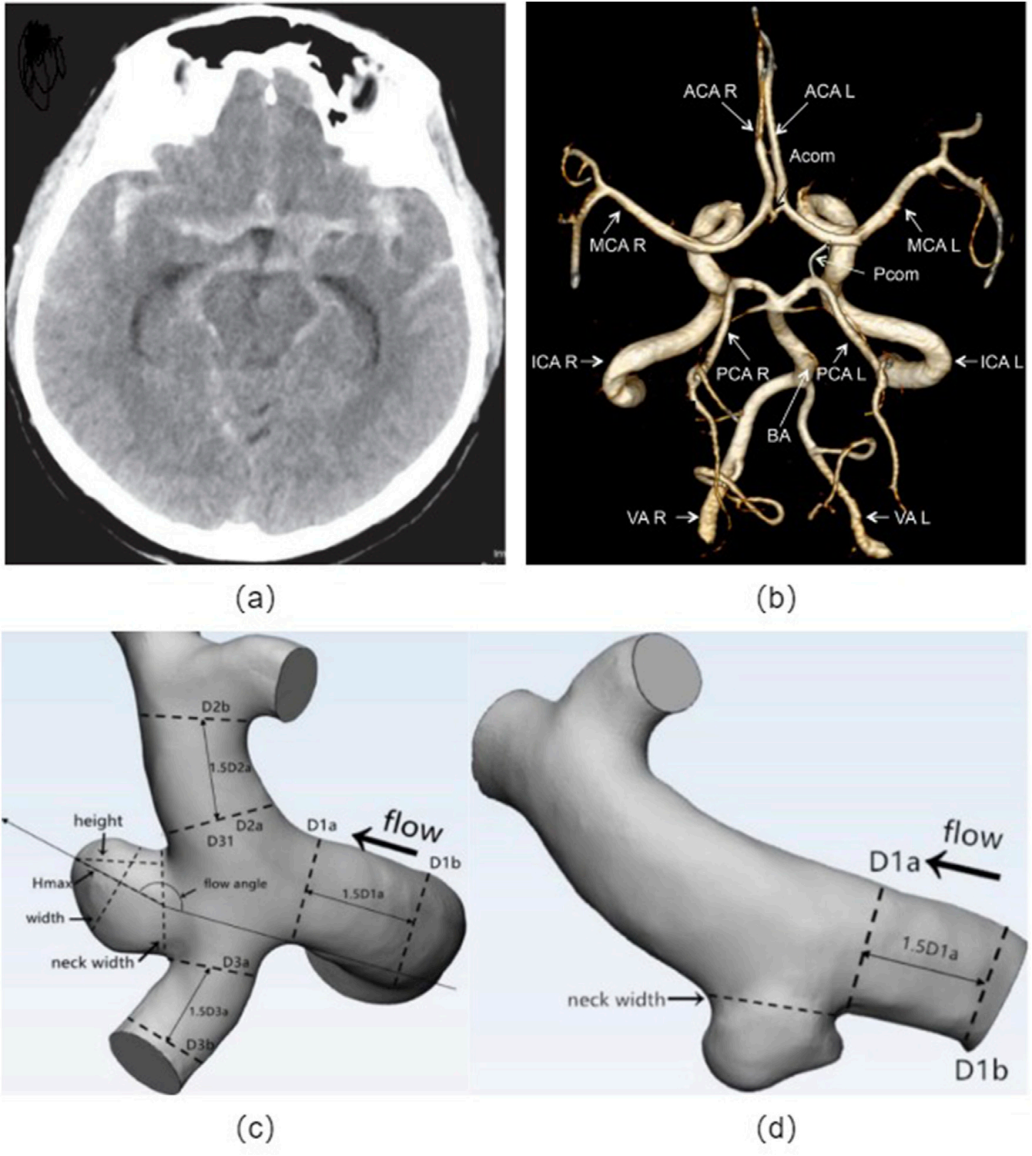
Cerebral artery morphology: (A) Non-contrast head CT showing subarachnoid hemorrhage from the left posterior communicating artery aneurysm; (B) Angiographic image of a human circle of Willis, indicated are: vertebral arteries (VA), right (R) and left (L), basilar artery (BA), internal carotid arteries (ICA R, ICA L), posterior cerebral arteries (PCA R, PCA L), anterior cerebral arteries (ACA R, ACA L), middle cerebral arteries (MCA R, MCA L), posterior communicating artery (Pcom) and anterior communicating artery (Acom); Definitions of morphological parameters in the bifurcation aneurysm (C) and sidewall aneurysm (D). H_max_: the maximum distance of the dome from the centroid of the neck plane, Height: the maximum perpendicular distance from the neck plane of the dome, Width: the maximum diameter perpendicular to H_max_, Neck width: the largest cross-sectional diameter of the aneurysm neck plane, aspect ratio (AR) equals to height/neck width, size ratio (SR) equals to H_max_/D_V_, and D_V_ denote the average of the diameter of the cross-sectional a vessel (Da) just proximal to the neck of the aneurysm and the diameter of the cross-section (D_b_) at 1.5 times Da from the neck of the aneurysm.
